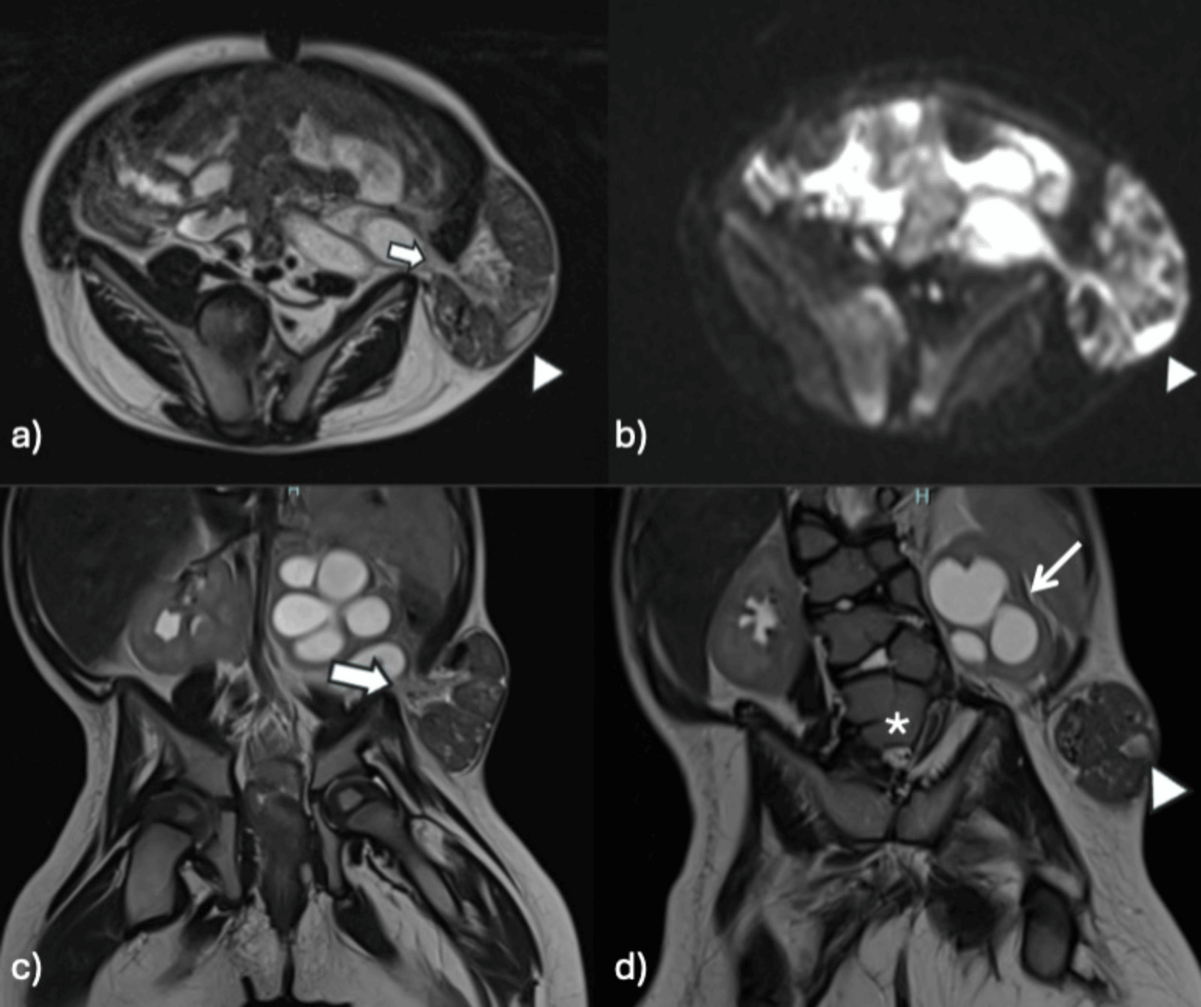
MRI axial and coronal sections (a) T2-weighted MRI, axial section, showing a defect (arrow) in the right lateral abdominal wall with the formation of a hernial sac containing intestinal contents, identifying an image inside compatible with a left testicle (arrowhead). (b) Diffusion-weighted MRI shows restricted diffusion of the left testicle (arrowhead). Although the corresponding ADC map demonstrated low signal intensity, confirming true diffusion restriction (not shown). (c) and (d) T2-weighted MRI in coronal sections, showing a defect (thick arrow) in the abdominal wall with the formation of a hernial sac containing intestinal contents, identifying a testicle inside (arrowhead). Additionally, previously known findings are noted, such as left hydronephrosis (thin arrow) and butterfly-shaped lumbar vertebral bodies (asterisk). ADC: apparent diffusion coefficient
